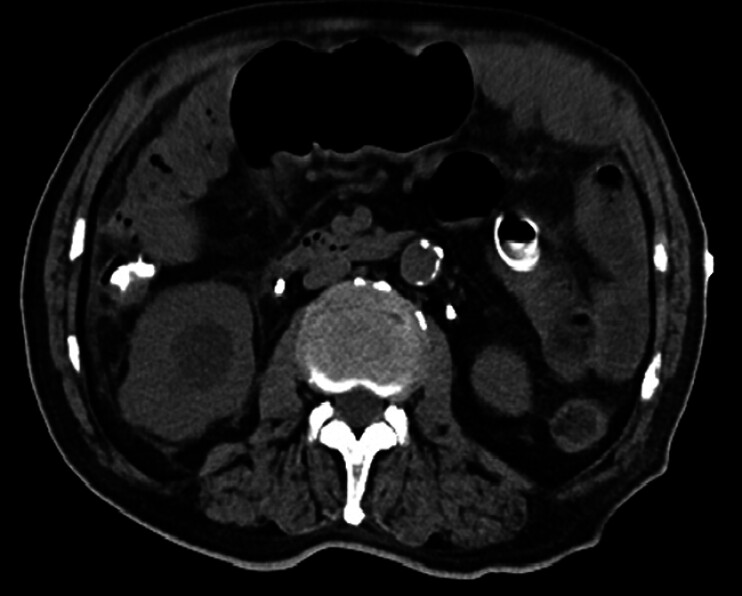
The follow-up abdominal CT imaging showed that the small bowel obstruction was significantly reduced. CT, computed tomography.
